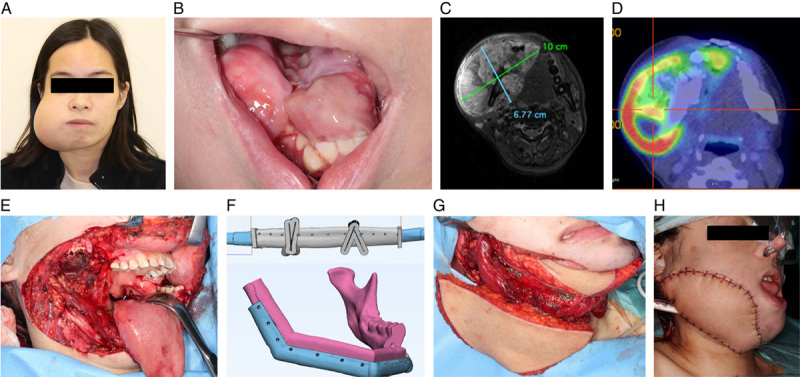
Chondroblastic osteosarcoma of mandible in a 39-year-old Chinese female. (A) Extraoral view; (B) Intraoral view; (C) Axial fat-saturated T2-weighted MRI image of the tumor; (D) PETCT scan of the tumor; (E) Defect after tumor resection and right partial mandibulectomy; (F) Virtual surgical plan of the fibula free flap reconstruction with osteotomy and positioning guides; (G) After inset of the fibula and antero-lateral thigh (ALT) free flaps; (H) After wound closure, ALT flap for extraoral skin defect and fibula free flap skin paddle for intraoral mucosal defect.
